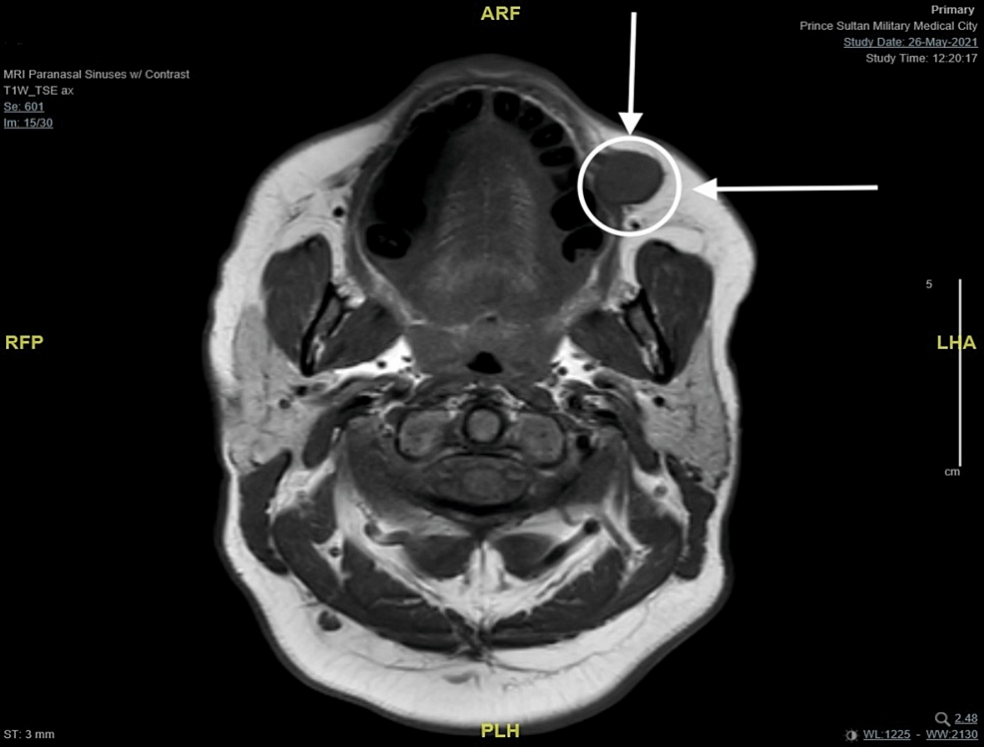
MRI T1-weighted image (axial cut) demonstrating iso-intense mass on the left buccal space. MRI: magnetic resonance imaging
